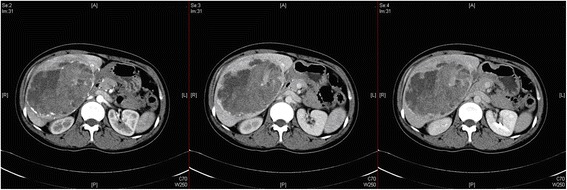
Upper abdominal dynamic enhancement computed tomography scan. Hepatic arterial enhancement is uneven, but the strengthening of the venous and balance periods is more even
